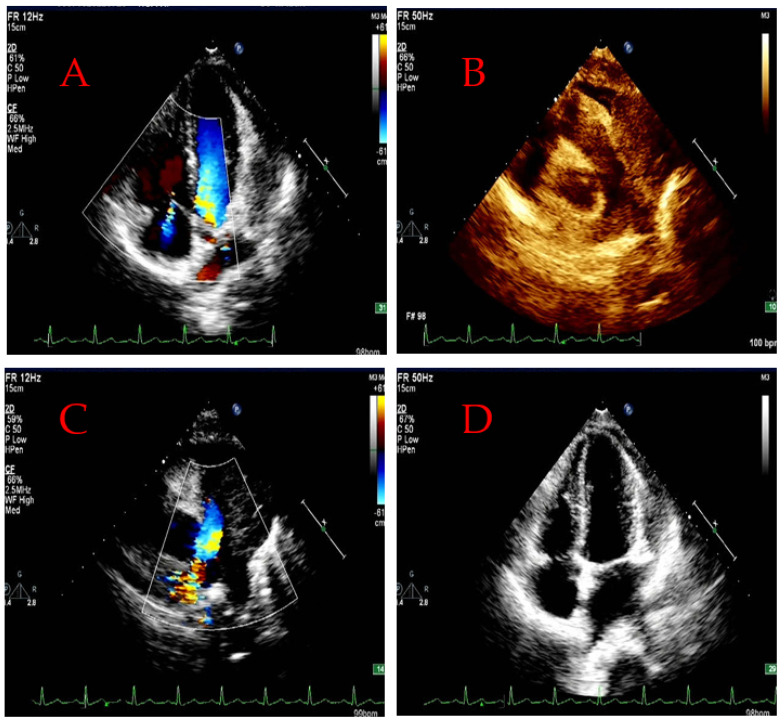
The patient’s echocardiography (A–D) (28 July 2022) showed that in the mediastinal and pulmonary artery masses tricuspid valve regurgitation (very mild), pulmonary hypertension (moderate), and biventricular systolic function were normal.
